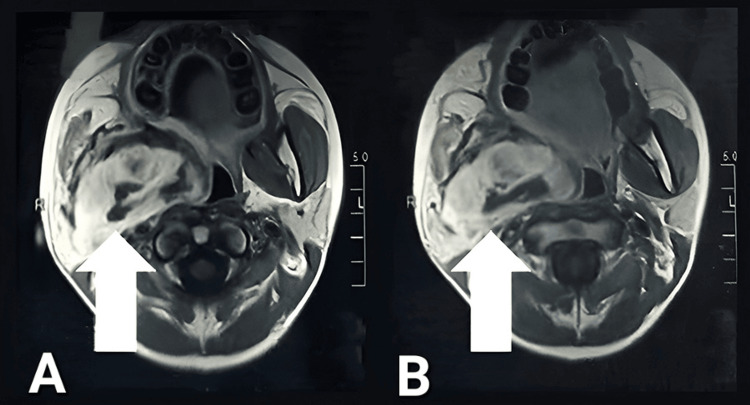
The MRI of the head showing a heterogeneous, lobulated soft tissue mass in the right parotid and masticator spaces, as marked by arrows in panels A and B.
